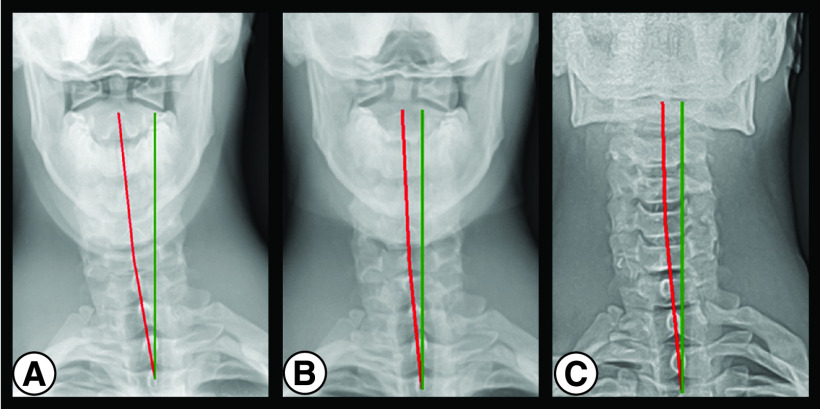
(A) Pretreatment, (B) post-treatment, and (C) 2-year follow-up anterior-to-posterior lower cervical radiographs.
